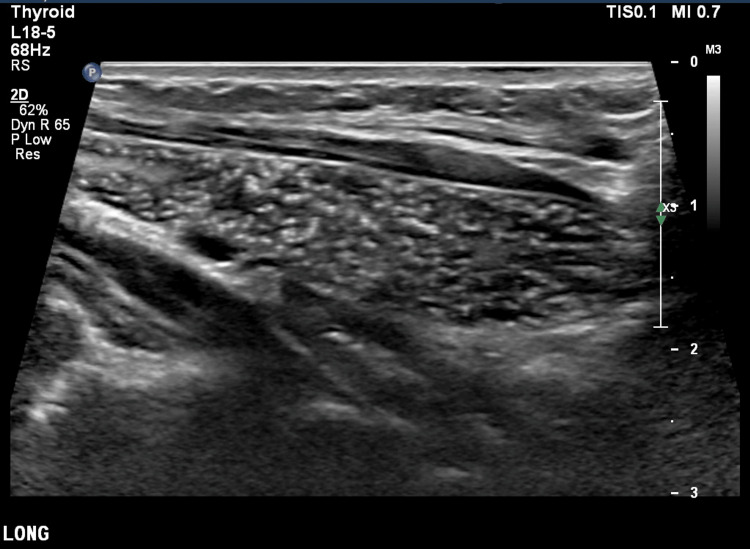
Longitudinal high-resolution ultrasound of the thymus demonstrating the characteristic starry-sky appearance due to multiple linear and punctate echogenic foci
